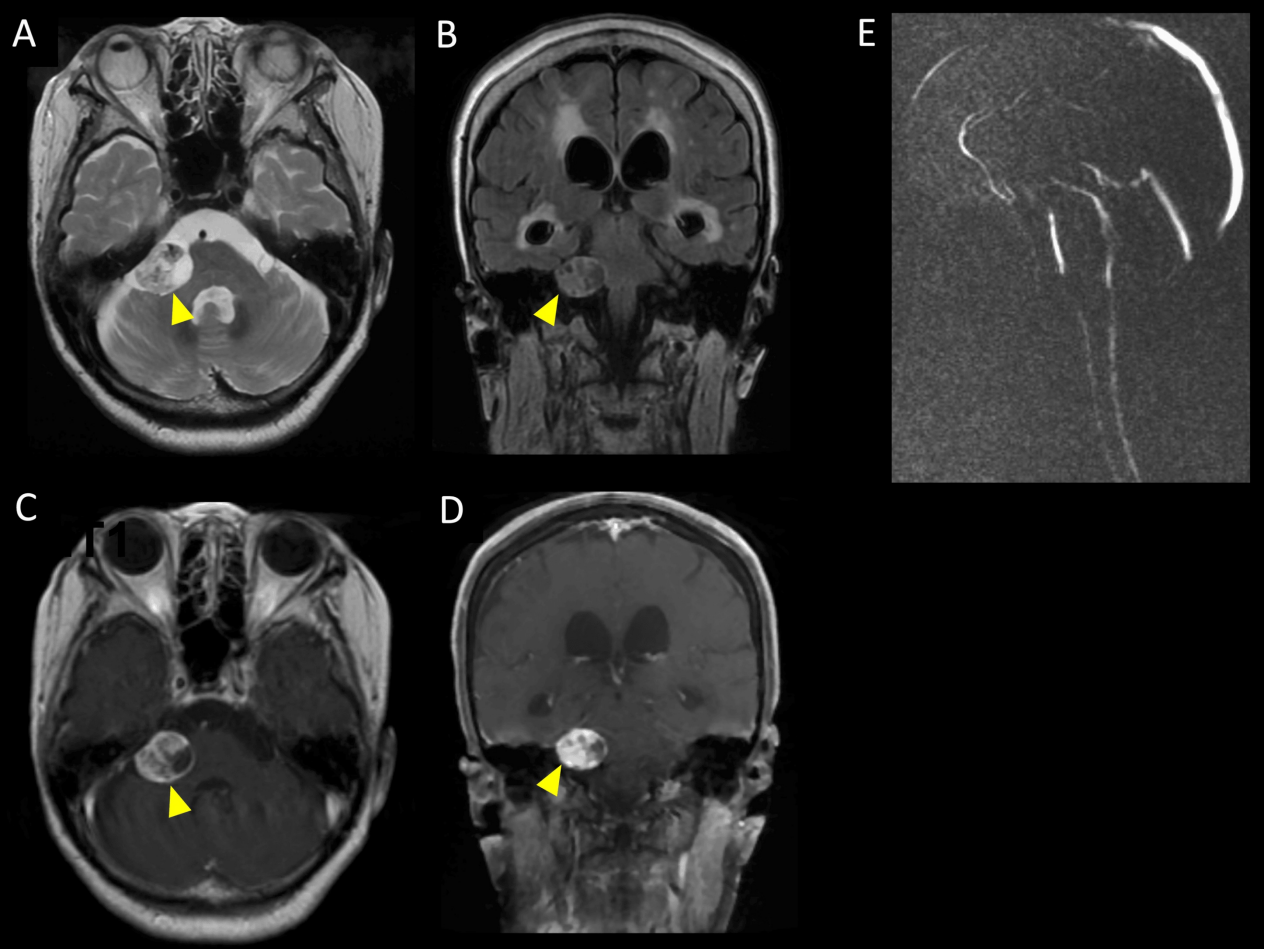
Brain MRI findings on admission VS: vestibular schwannoma; CSF: cerebrospinal fluid; MRI: magnetic resonance imaging; T2WI: T2-weighted imaging; FLAIR: fluid-attenuated inversion recovery (A) Brain MRI reveals a 22 mm VS with a mixture of moderately high- and low-intensity signals in the right cerebellopontine angle on axial T2WI (a yellow arrowhead). (B) The coronal FLAIR image shows that the tumor is compressing the brainstem to the left. (C, D) Gadolinium-enhanced T1-weighted imaging shows enhancement of the tumor and ventricular enlargement (a yellow arrowhead). (E) Cine MRI shows CSF flow from the fourth ventricle through the cerebral aqueduct into the third ventricle
